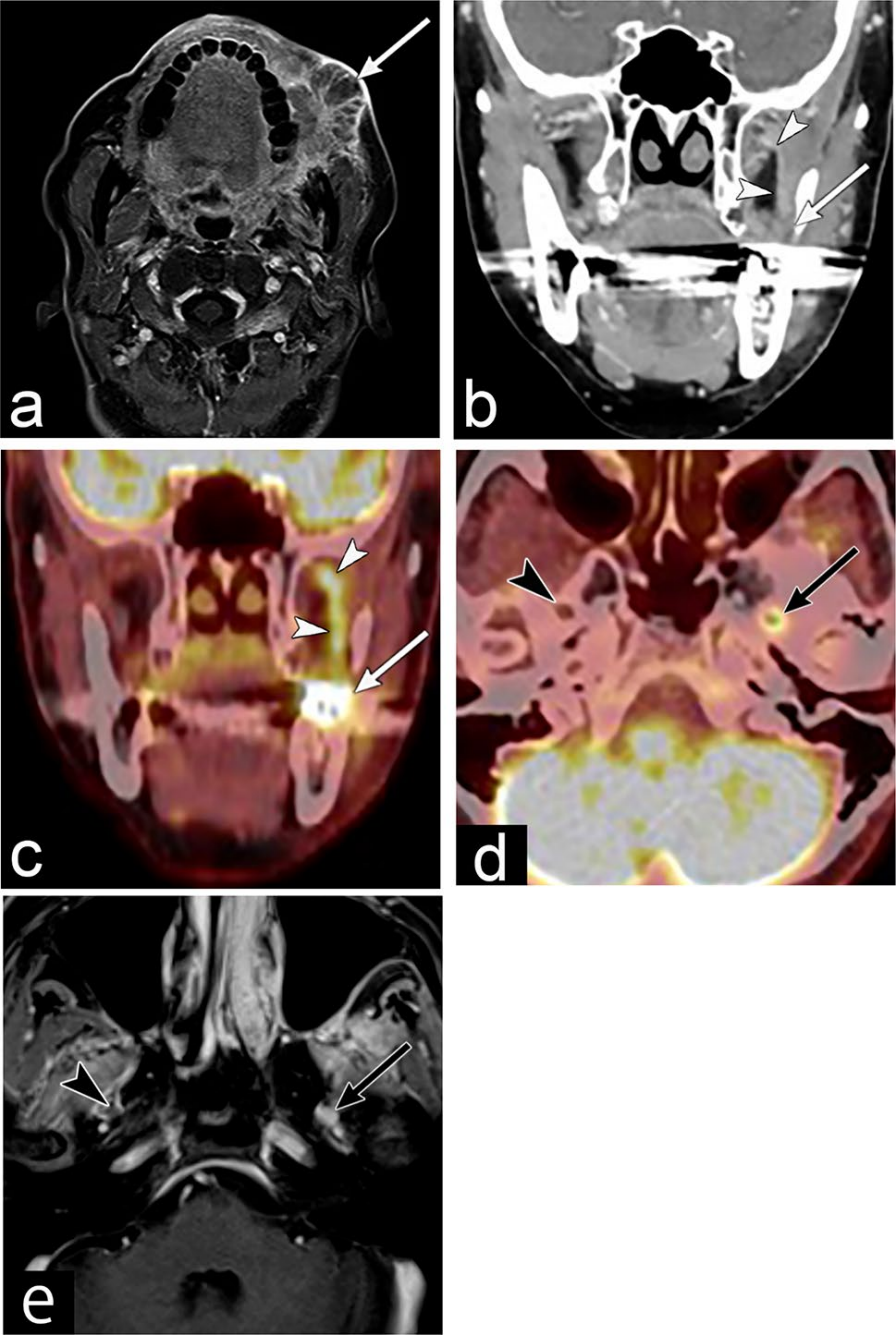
Perineural spread of buccal mucosa squamous cell carcinoma of a woman in her 50s with left buccal swelling. a Axial contrast-enhanced (CE) T1 weighted images (WI) with fat suppression show enhanced mass (white arrow) in the left buccal space. b–d 18F-fluorodeoxyglucose (FDG) positron emission tomography CE CT shows linear enhancement and increased FDG uptake (white arrowheads) extending upward from the left buccal mucosa mass (white arrow). This is continuous within the left foramen ovale, and shows increased FDG uptake (black arrow) within the left foramen ovale. The black arrowhead indicates a normal right foramen ovale. e Axial CE T1WI with fat suppression shows enhanced mass within the left foramen ovale (black arrow). The black arrowhead indicates a normal right foramen ovale
